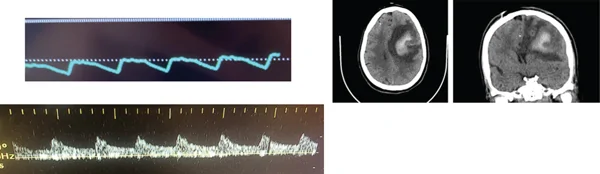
Normalization of the ICP waveform with p1 > p2 > p3. Recovery of the middle cerebral artery diastolic flow after aggressive medical therapy (TCCD, inferior panel). CT scan demonstrated natural evolution of the hemorrhage with reduction of the hematoma size.
